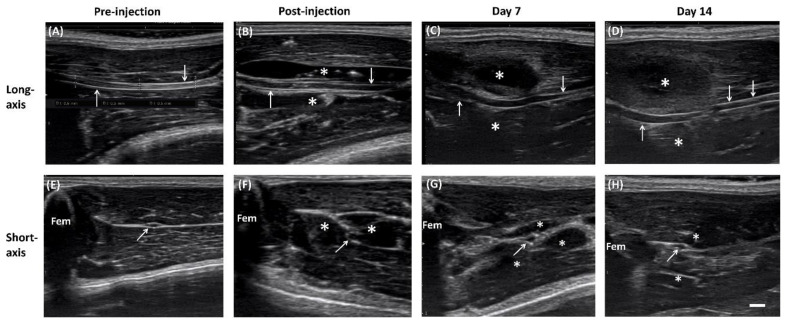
Sonography of sciatic nerve before and after injection. Long-axis (A–D) and short-axis (E–H) views of the sciatic nerve (arrows) before, immediately after, and 7 days and 14 days after hydrogel (asterisk) injection (Scale bar: 1 mm).
